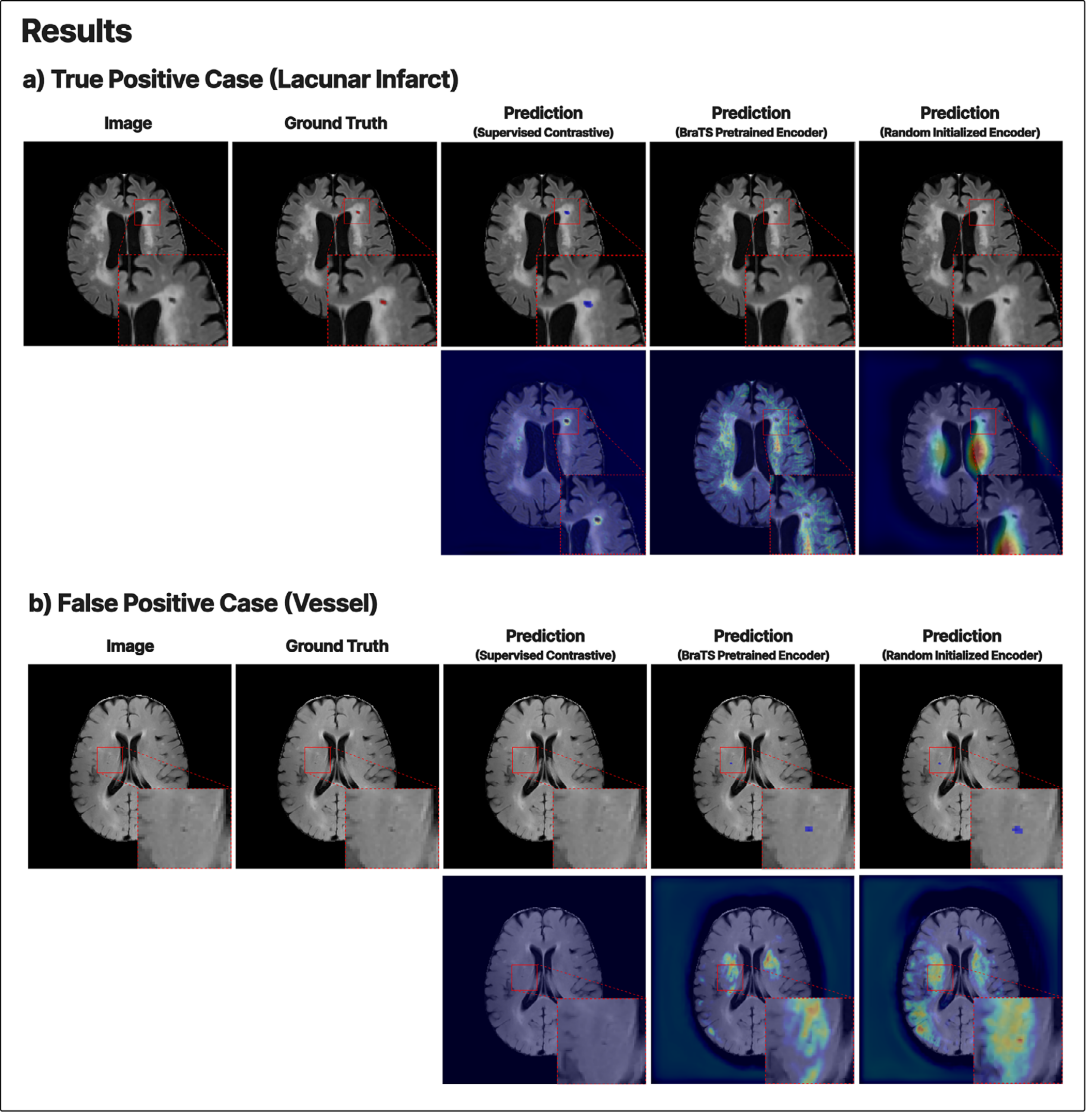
# Discriminative Feature Learning for Lacune Detection in 2D T2‐FLAIR Images using Supervised Contrastive Learning
